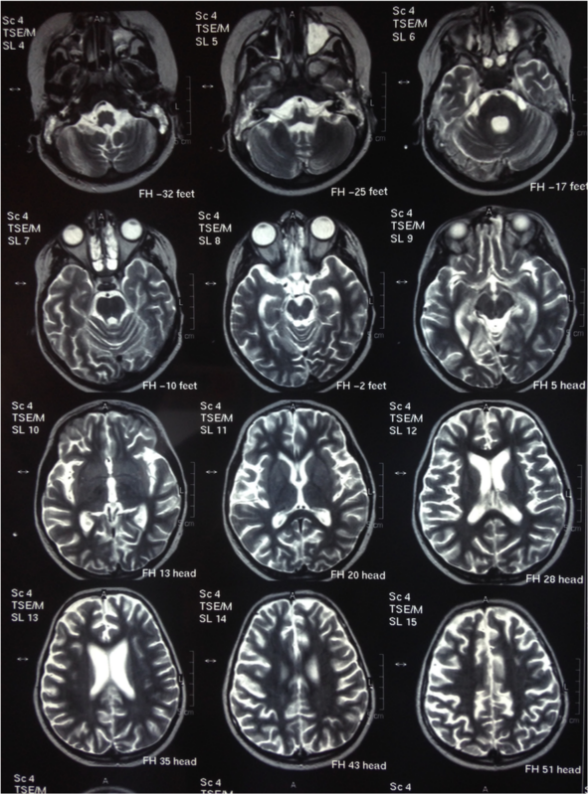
Brain magnetic resonance imaging sections showing generalized brain atrophy
